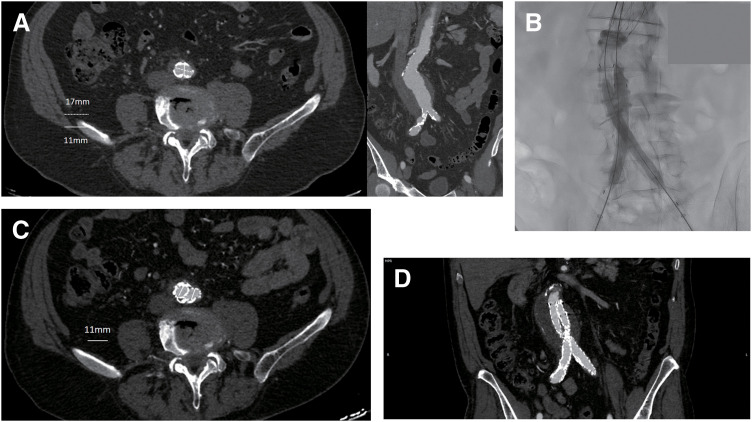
Fig. 1 (A) Axial and coronal views of a preoperative CT of a patient with a narrow aortic bifurcation. (B) Intraoperative image of simultaneous deployment of iliac limbs and kissing ballooning. (C) Postoperative CT image indicating successful expansion of iliac limbs at the level of the aortic bifurcation. (D) Postoperative coronal view indicating successful deployment of the limbs. CT: computed tomography
